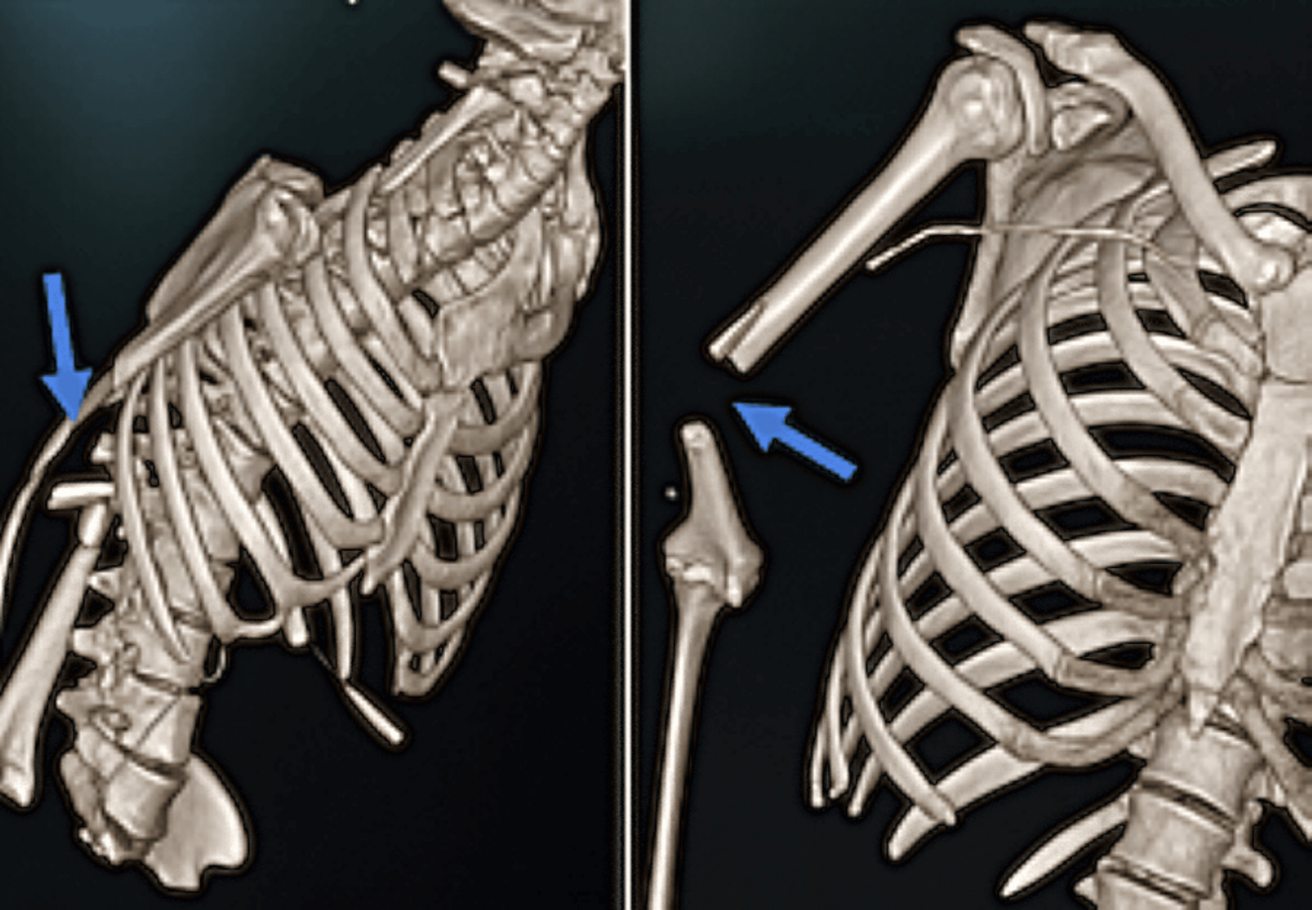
Three-dimensional reconstruction from a CT scan of the right upper limb showing an open diaphyseal fracture of the distal third of the humerus, characterized by a transverse fracture line and marked anterolateral displacement of the fracture fragments (blue arrows).
